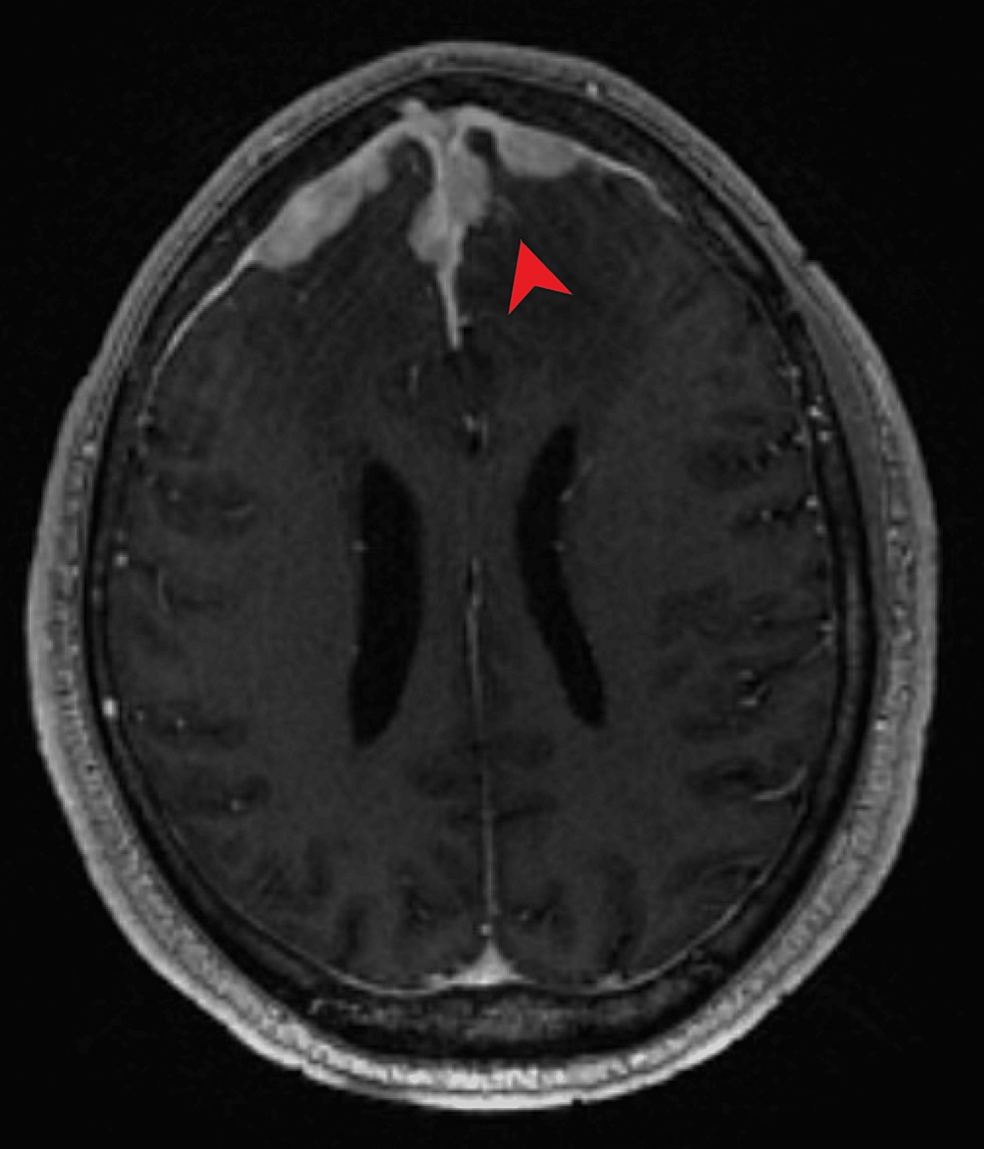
Axial T1-weighted MRI of the brain with contrast showing extensive enhancing dural nodularity along bilateral frontal convexities and anterior falx (red arrow)
